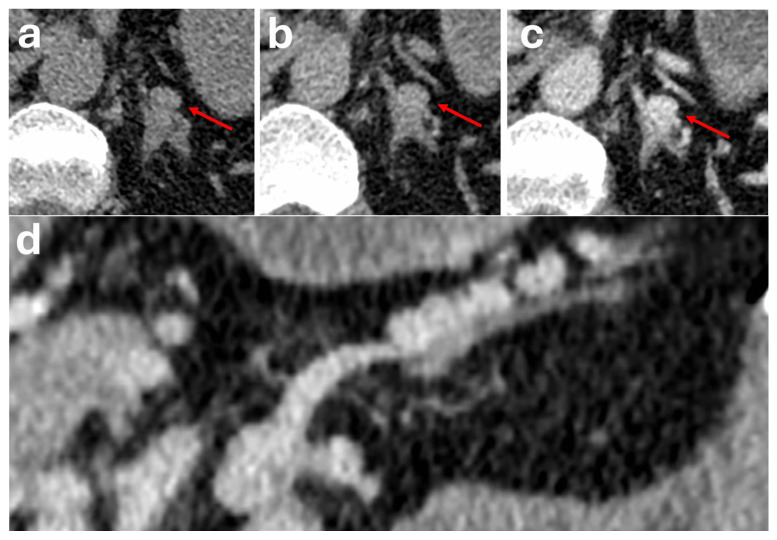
Patient with an incidental 1.3 cm left adrenal nodule measuring 44 HU on unenhanced CT thorax (red arrows) (a). The nodule measured 145 HU on portal venous phase (b) and 65 HU on delayed phase (c). Absolute washout is 79.2%. Relative washout is 55.2%. On multiplanar reformat, the nodule was noted to be part of a serpiginous structure that enhanced in tandem with adjacent vessels and demonstrates continuation with a tributary of the left renal vein (d). Overall features are in keeping with an adrenal varix. The patient remained asymptomatic with no evidence of liver cirrhosis and a normal liver Fibroscan result.
